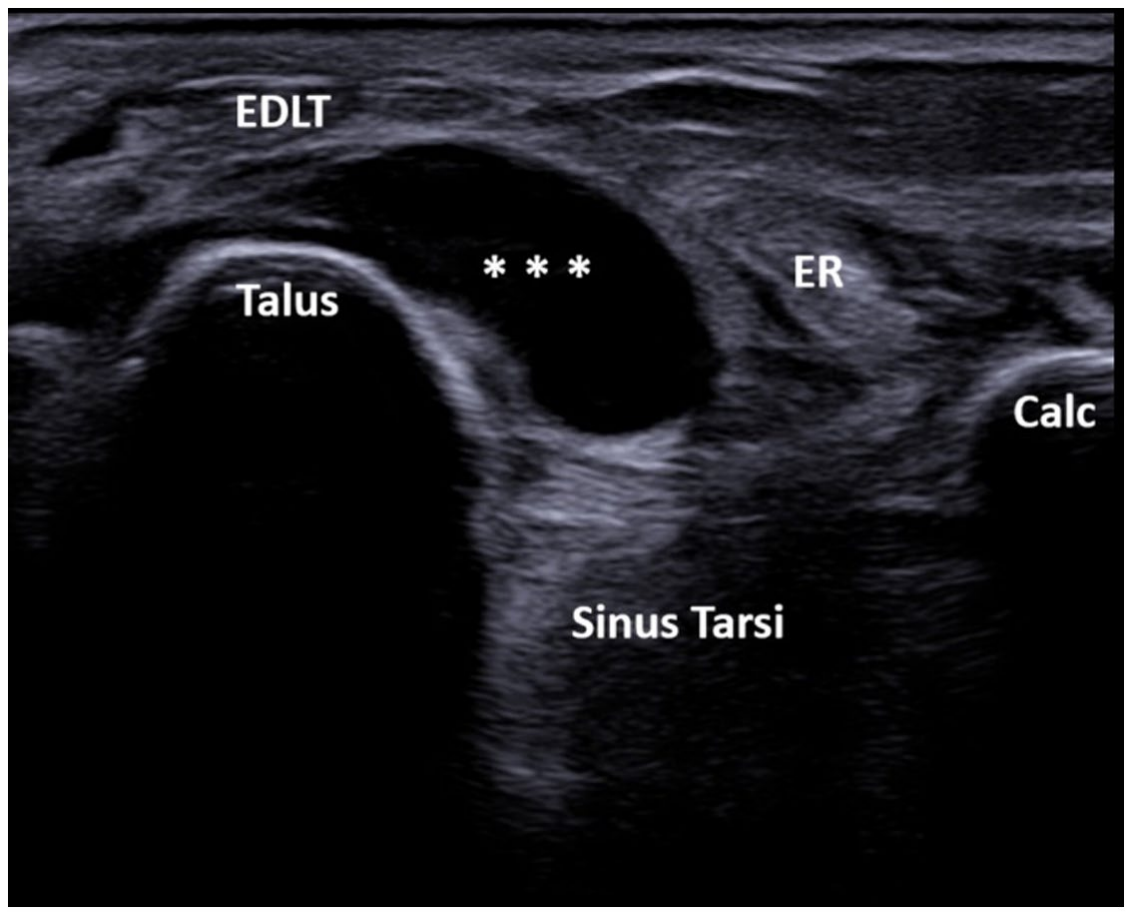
A 60-year-old patient with systemic sclerosis. Ultrasound image showing unilocular GB with the transducer positioned in the short axis of the foot (left side is medial). The transverse ultrasound image of the dorsolateral ankle shows an anechoic unilocular fluid collection (*) between the extensor digitorum longus tendon (EDLT) and the talus. ER, extensor retinaculum; Calc, calcaneus; and EDLT, extensor digitorum longus tendon.
